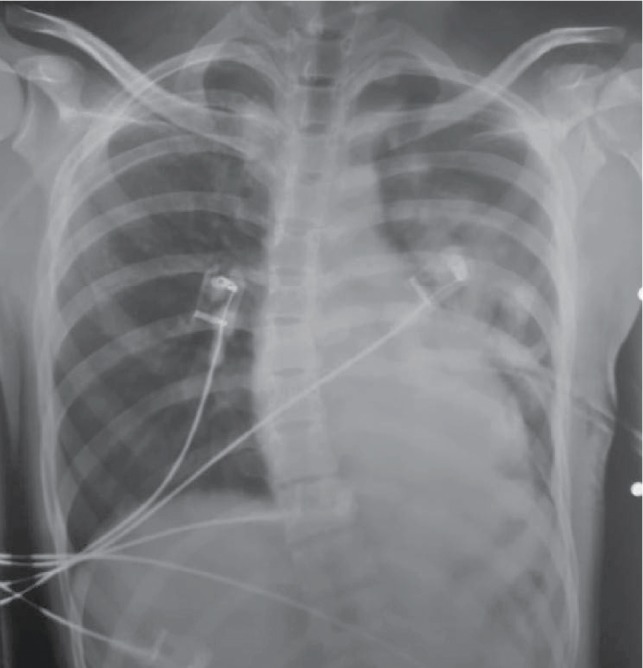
Chest X-ray revealed satisfactory pulmonary expansion after thoracic tube insertion.
